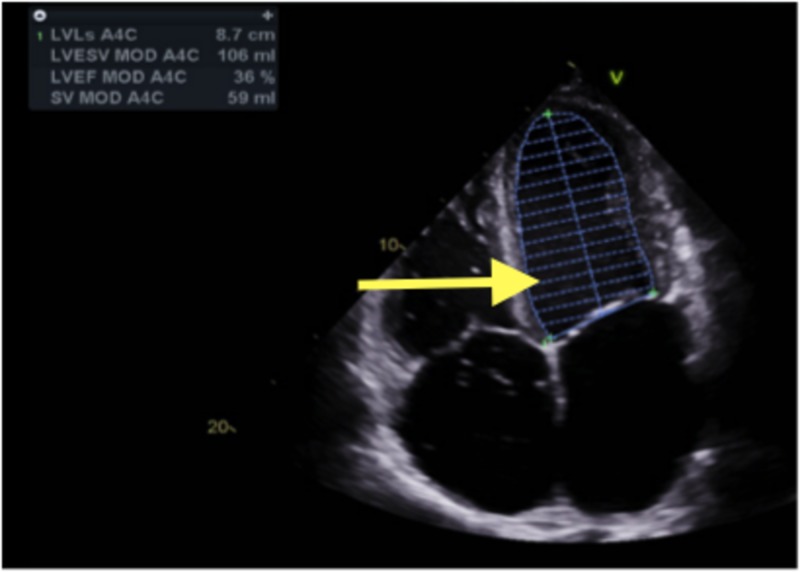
Echocardiogram: Arrow pointing toward dilated left ventricle
